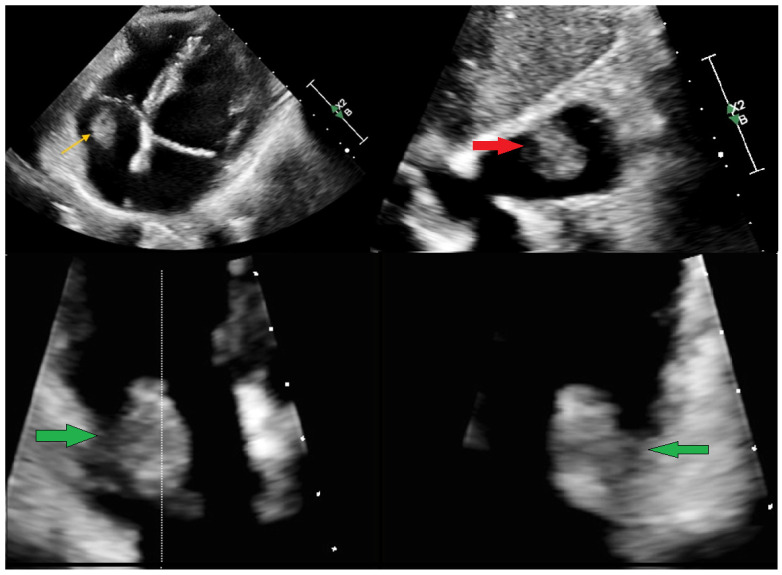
A 51-year-old female patient with a left-sided breast carcinoma after neoadjuvant cycles of chemotherapy was referred to the Emergency Department (ED) with a transthoracic echocardiography (TTE) describing an ambiguous structure located in the right atrium (RA). Initially, two months before the admission, the lesion was presumed to be of thrombotic origin. Despite prior anticoagulation therapy with nadroparin, later switched to enoxaparin, no regression of the atrial mass was observed. Referred to the ED, the patient was asymptomatic, with an unremarkable physical examination and no signs of heart failure, chest pain, syncope, or systemic inflammation. The results of routine biochemical and haematological investigations were within normal limits. The patient presented with an indwelling vascular access chemotherapy port. Repeated TTE (above) revealed a well-defined, hyperechoic, mobile mass of 12 × 19 mm within the RA. The lesion was attached to the atrial wall directly beneath the tricuspid valve, prolapsing through the valve orifice without evidence of flow obstruction. No abnormal echogenicity was identified around the vascular access port, and no vegetation or features suggestive of infective endocarditis were present. Global cardiac contractility was preserved, with no regional wall motion abnormalities. Yellow arrow—RA mass in apical 4-chamber view. Red arrow—RA mass in substernal 4-chamber view. Green arrow—RA mass in apical 2/4 chamber view by X-plane. The video recording can be found in Supplement S1–S3.
